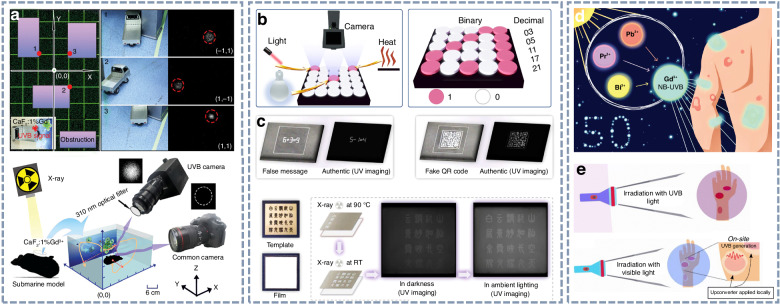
Potential applications of UVB light. a Static and dynamic tagging application of the X-ray-irradiated CaF_2_:Gd^3+^ phosphor. Reproduced with permission from ref. ^108^. Copyright 2024 John Wiley & Sons, Inc. b Optical information storage application of the X-ray-irradiated ScBO_3_:Bi^3+^ phosphor under light and heat stimulus. Reproduced with permission from ref. ^101^. Copyright 2024 Springer Nature. c Anti-counterfeiting application of the X-ray-irradiated NaYF_4_:Gd^3+^ phosphor under indoor lighting conditions. Reproduced with permission from ref. ^105^. Copyright 2025 John Wiley & Sons, Inc. d UVB phototherapy concept of the 254 nm-irradiated Sr_3_Gd_2_Si_6_O_18_:Pr^3+^ phosphor for small-area skin disorders. Reproduced with permission from ref. ^103^. Copyright 2021 Royal Society of Chemistry. e On-site UVB phototherapy application of the NaYF_4_:Ho^3+^, Gd^3+^ upconversion phosphor. Reproduced with permission from ref. ^180^. Copyright 2024 Royal Society of Chemistry
